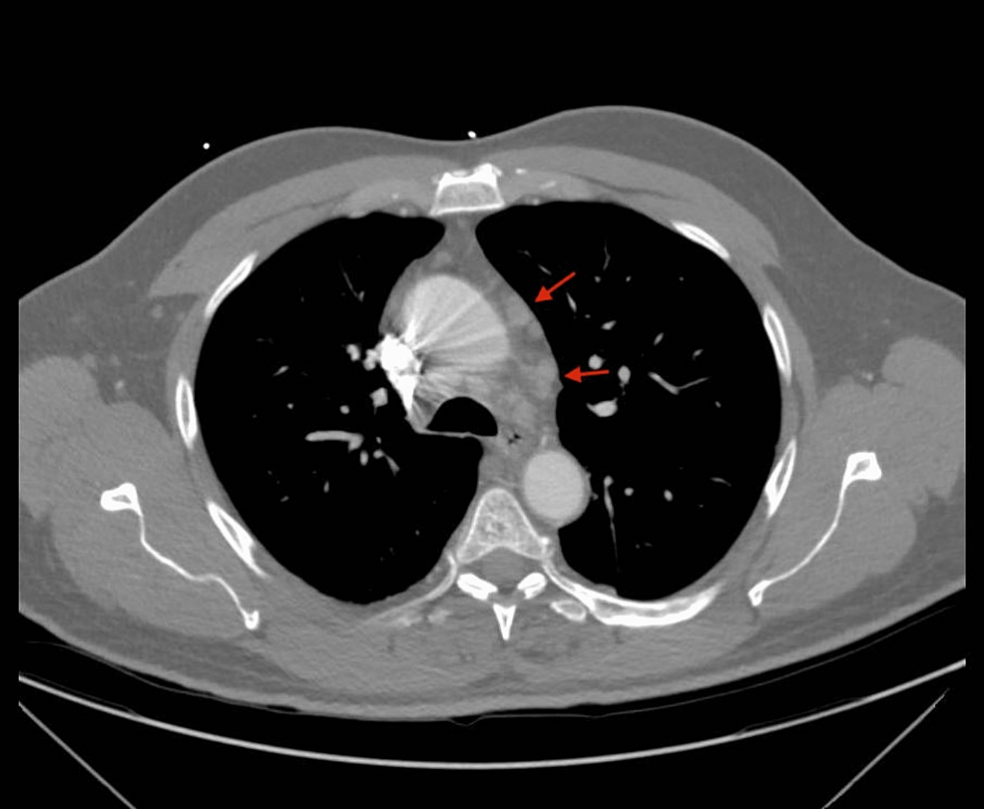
Initial CTA of chest showing mediastinal lymph nodes (red arrows) CTA: CT angiography
